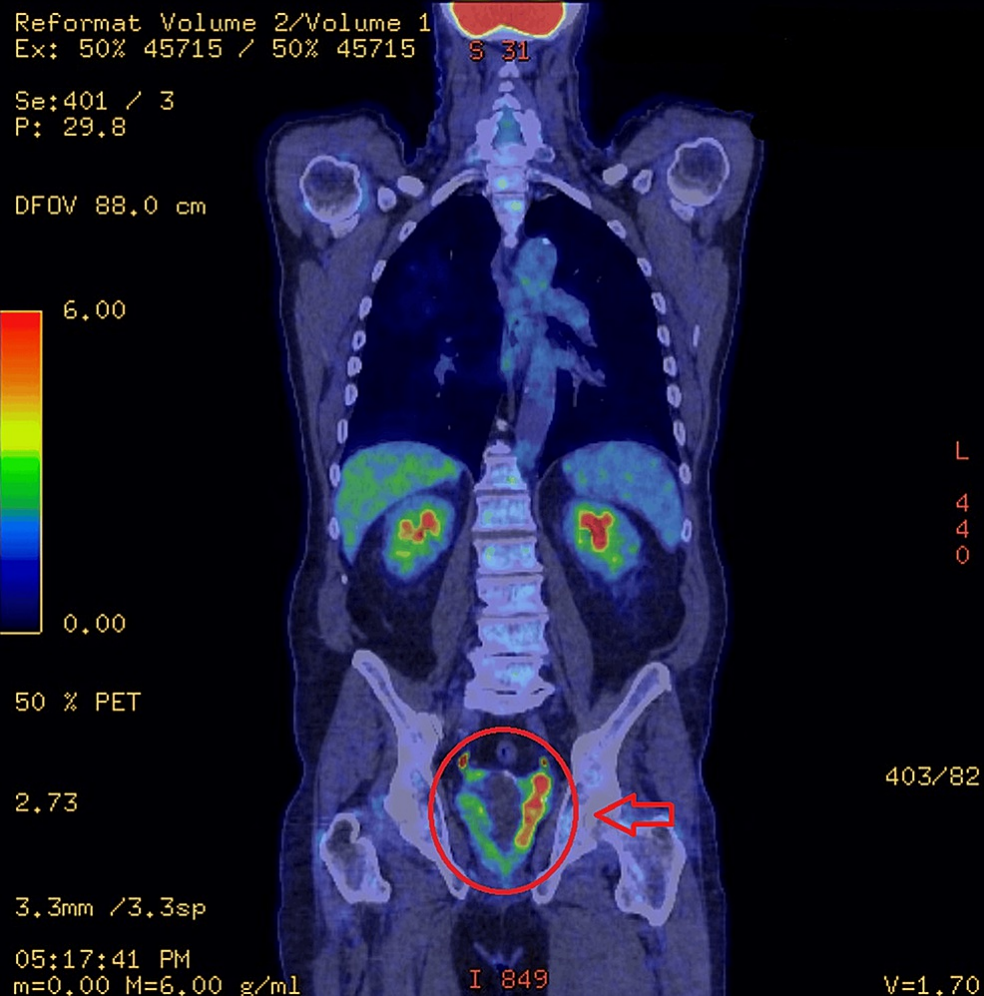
An FDG PET-CT image of the body (notice FDG uptake in prostate and seminal vesicles)
